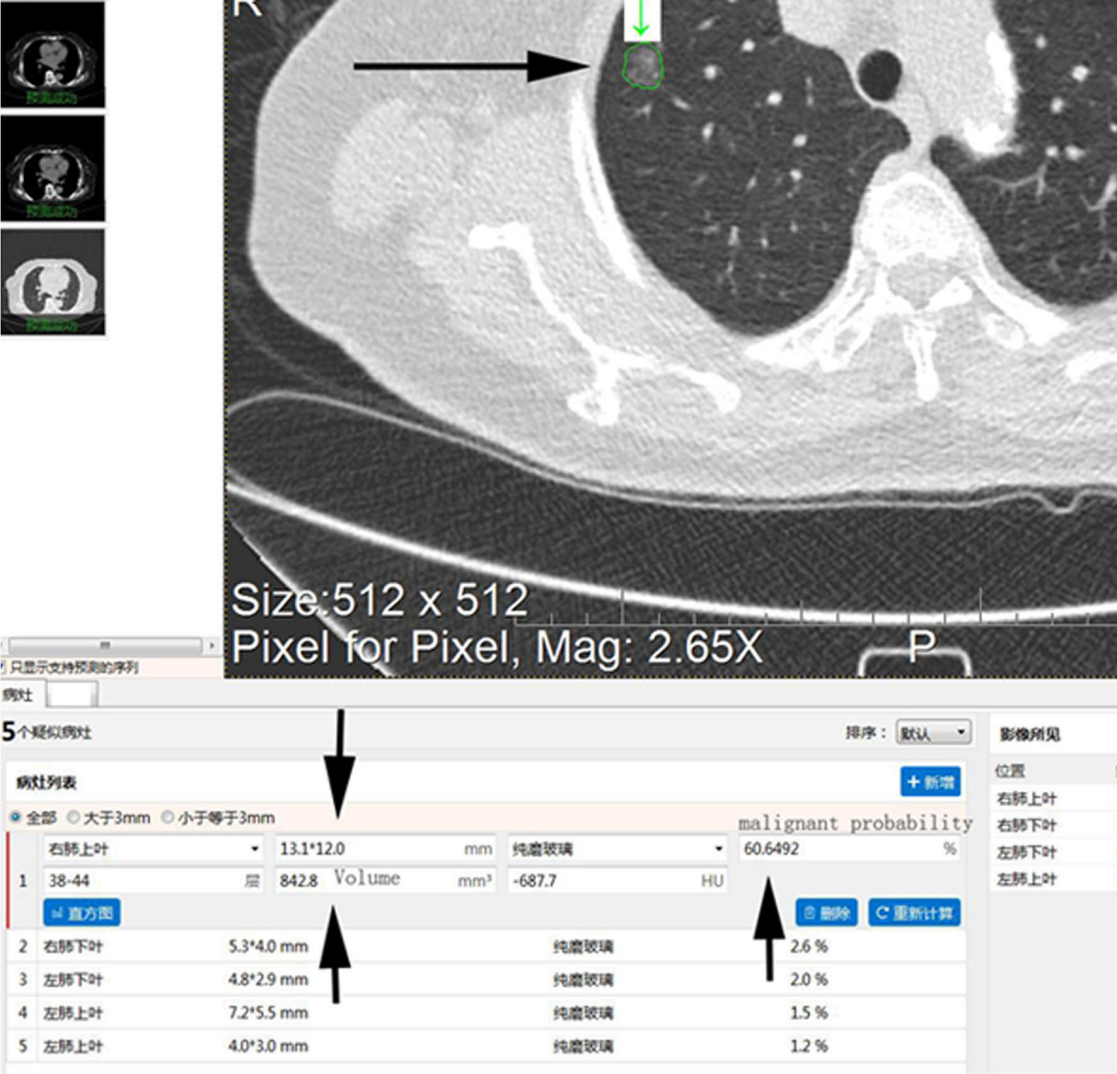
Screenshot of AI-assisted diagnostic software in the analysis of a specific CT image. The long arrow shows the analysis of an LDCT image in which all detected nodules have been marked. The short arrow indicates detailed information regarding the underlying nodule (e.g., location, maximum diameter, minimum diameter, density type, malignancy probability, volume, mean CT value). AI, artificial intelligence; LDCT, low-dose computed tomography; CT, computed tomography.
